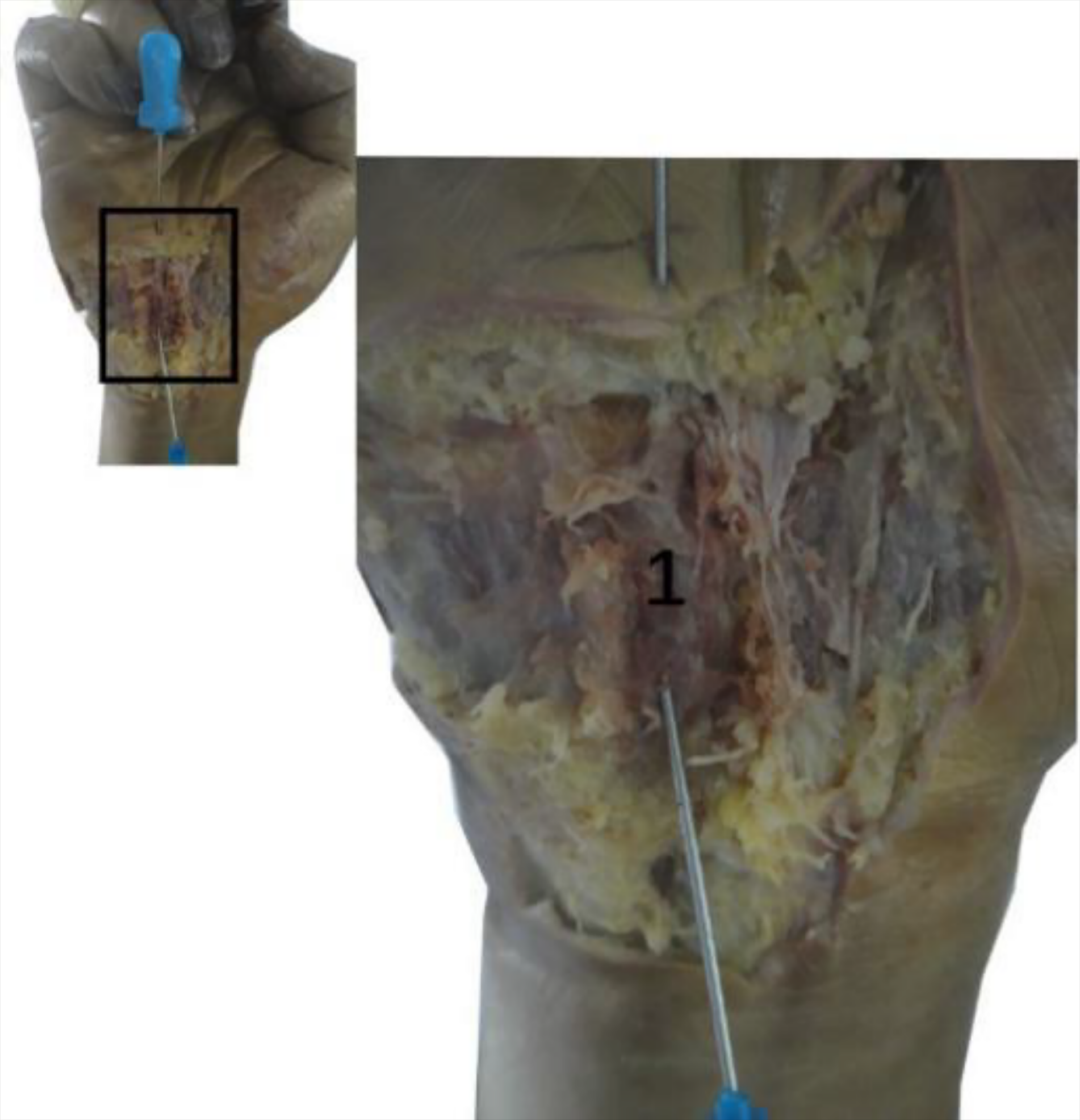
The needle knife penetrated the ulnar artery in the non-ultrasound guided group. 1, Ulnar artery.
